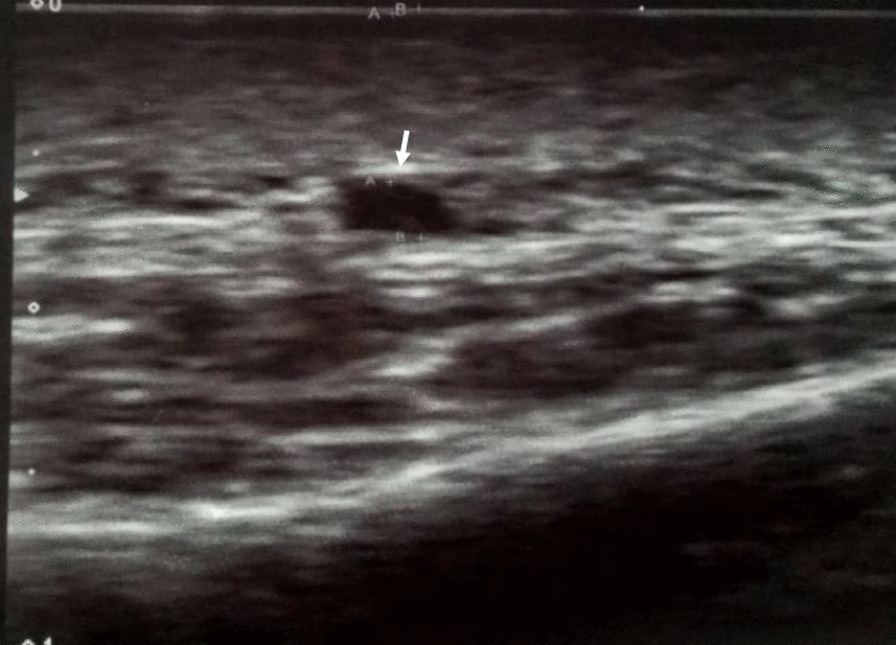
Cross-sectional Doppler ultrasound view of right temporal artery. Arrow is pointing to hypoechogenic halo sign
